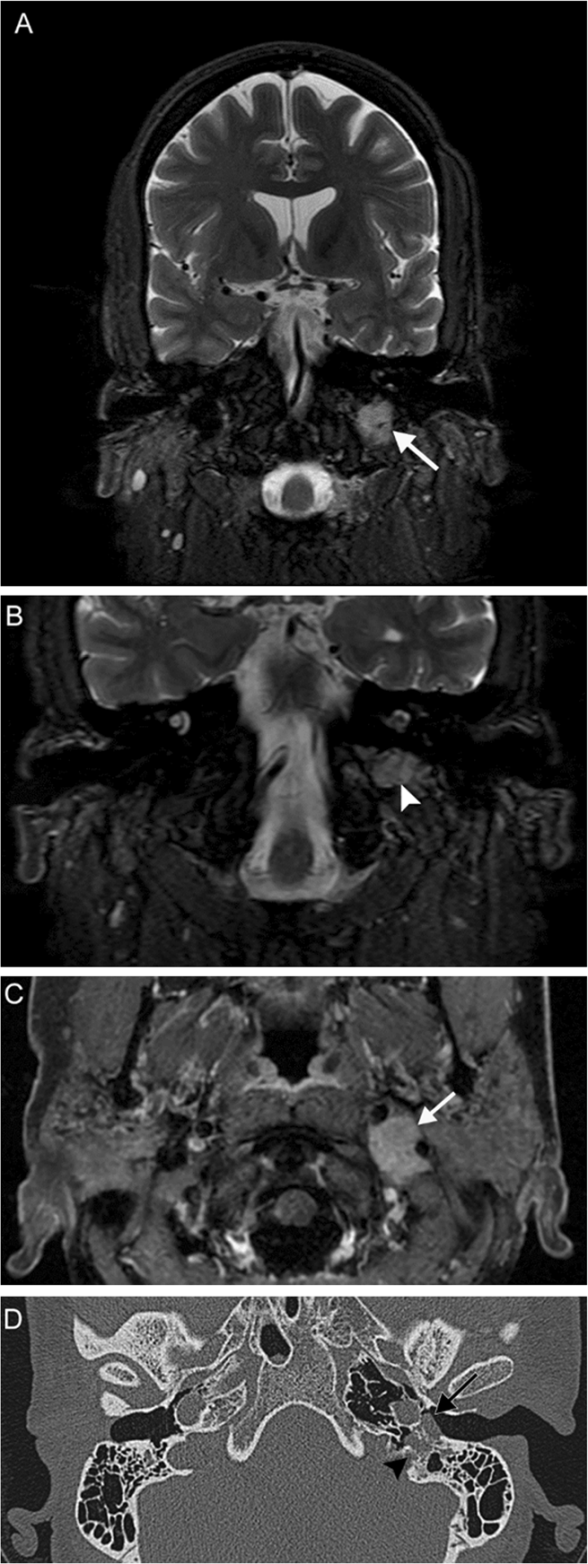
Jugulotympanicum paraganglioma. A 49-year-old woman with voice change and pulsatile tinnitus. Coronal T2-weighted MR images (a, b) and axial contrast-enhanced T1 image (c) demonstrate a T2-hyperintense, enhancing mass centered at the left jugular bulb (white arrows) extending into the hypotympanum (white arrowhead). Axial CT of the temporal bones (d) reveals abnormal soft tissue in the left middle ear (black arrow) with osseous 'moth-eaten' destruction at the jugular foramen (black arrowhead); the cochlear promontory in preserved (not shown). Based on location and appearance, findings are consistent with a jugulotympanicum paraganglioma (glomus jugulotympanicum tumor)
